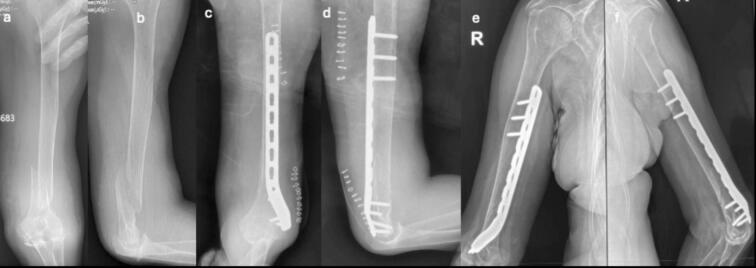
Case 4 – Female patient, 69 years old, mechanism of injury: fall from standing height. a-b) Preoperative AP and lateral radiographs showing AO 1.2.A.1-c fracture; c-d) Immediate postoperative AP and lateral radiographs; e-f) AP and lateral radiographs at 1-month follow-up.
